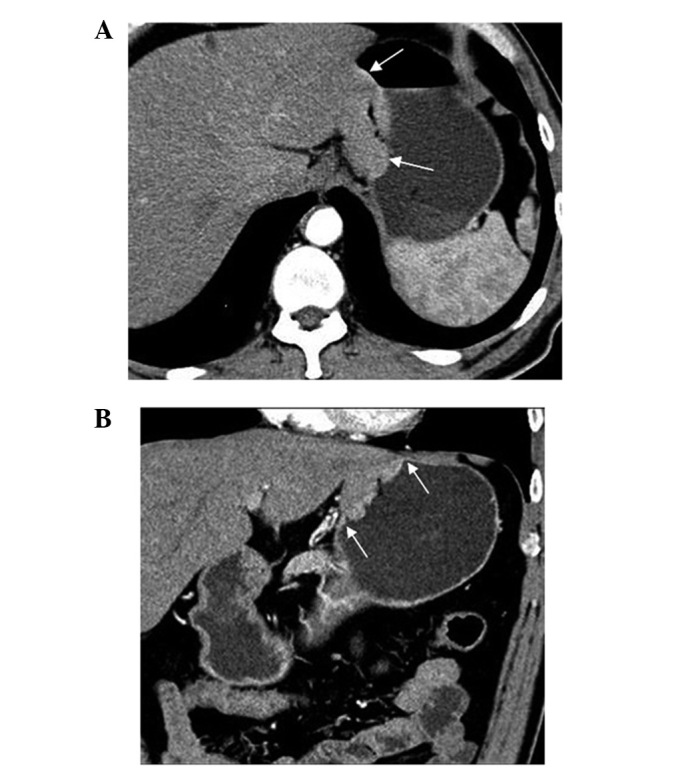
Contrast-enhanced CT scan and coronal reformatted images showed (A) a well-circumscribed mass in the lesser curvature with a large thickness-to-width ratio (arrows). (B) The low-density stripe of the normal gastric wall abruptly terminated at the edge of the lesion (arrows). The majority of the lesion was located in the submucosa according to pathological investigation.
